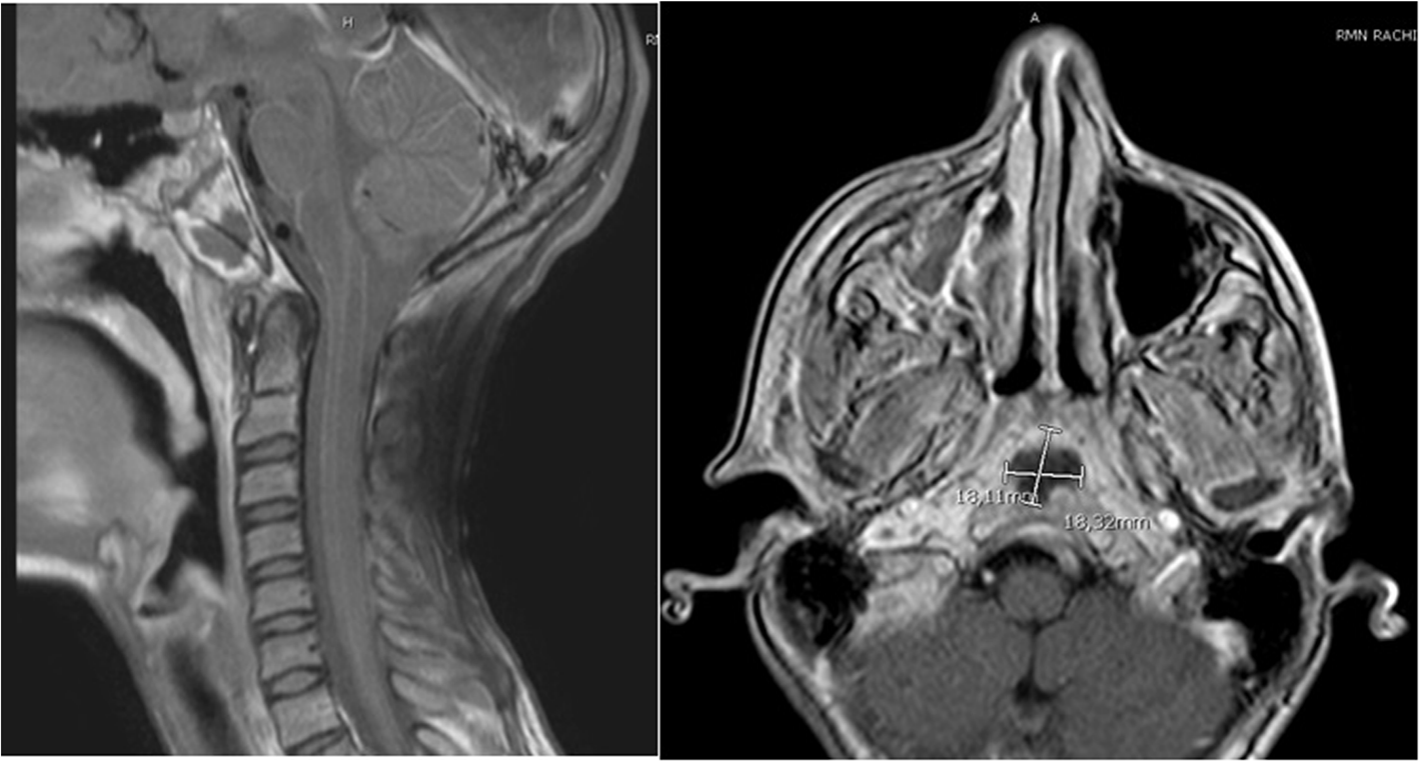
Axial and sagittal MR images of abscess of the clivus
